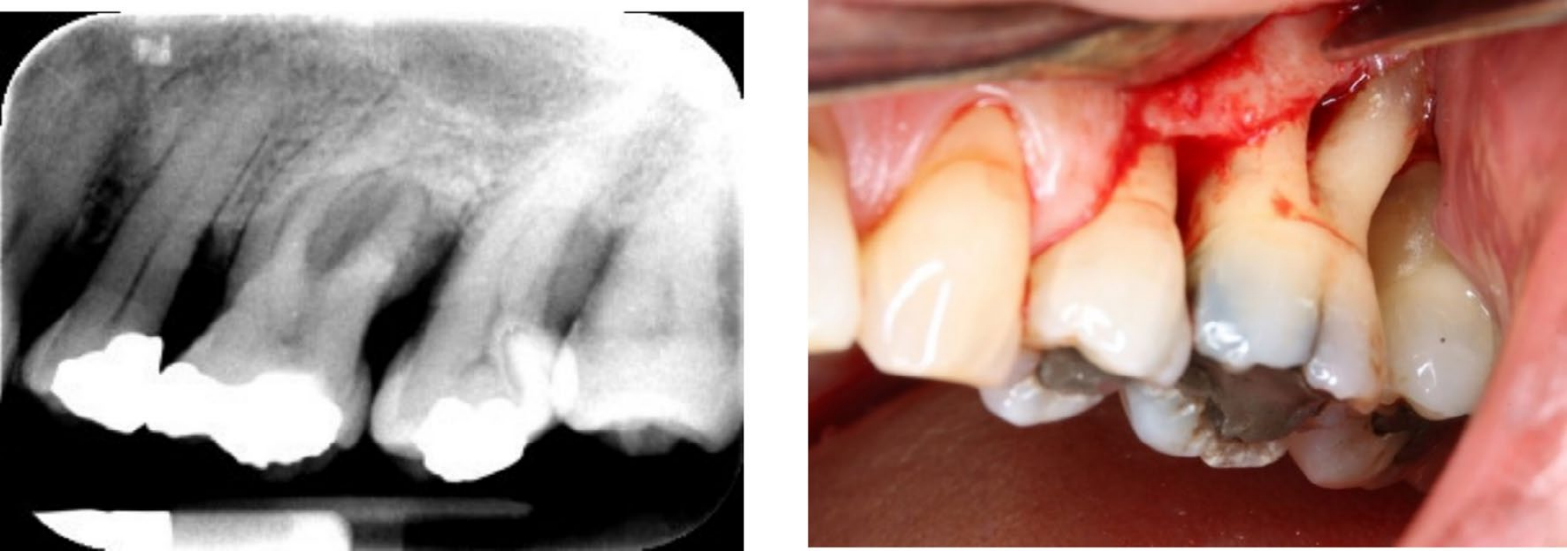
(a) Pre‐operative periapical radiograph of UL6 at baseline. (b) Intra‐operative photo of UL6 which was randomized to NSPT for treatment 1 and following a lack of success at the 6‐month review, was randomized to OFD for treatment 2.
